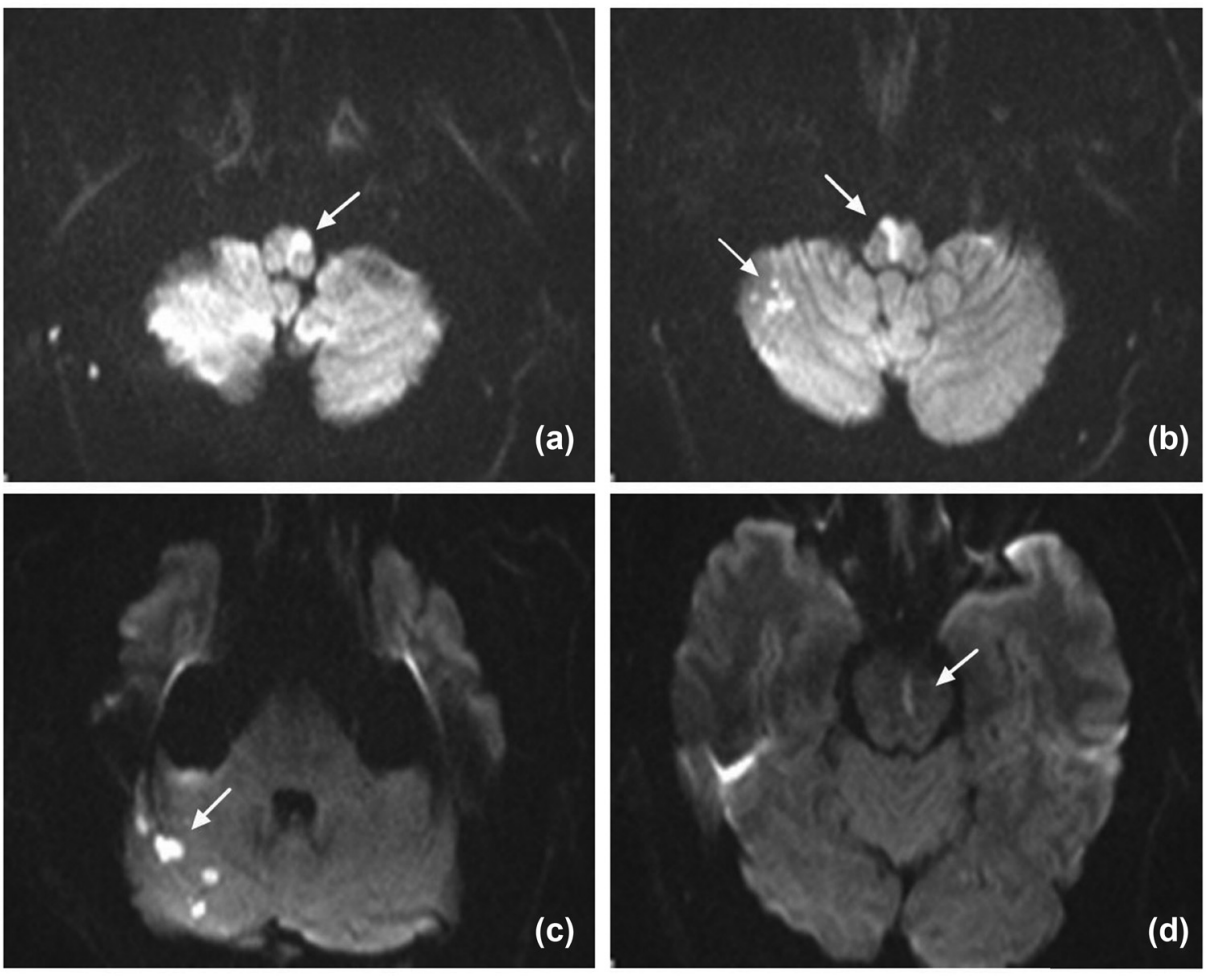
(Axial DWI imaging): (a and b; arrow) bilateral medial medullary infarction involves the ventral, middle parts of the medulla oblongata, which were compatible with atypical “heart appearance.” (b and c) The right cerebellar hemisphere and (d) pons also showed high-intensity lesions.
